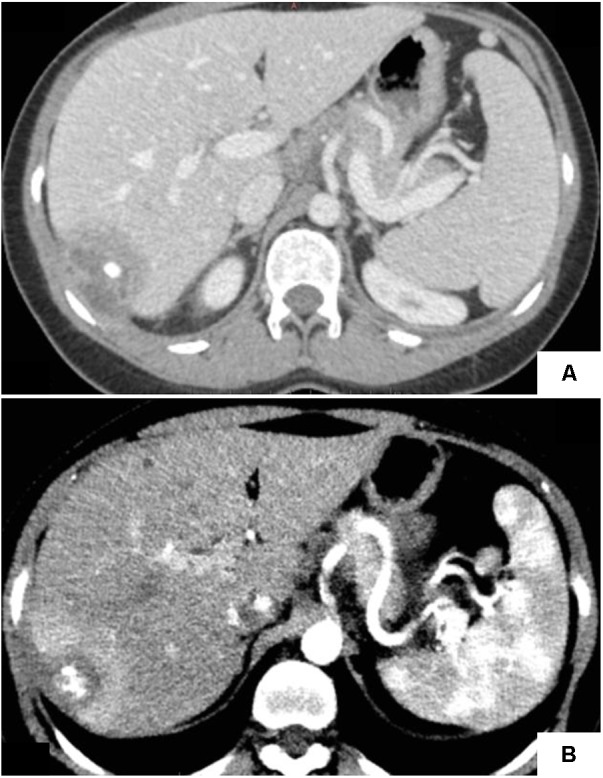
Abdominal CT-scans showing liver abscess. (A) Central calcification of the liver abscess observed in 2010, suggesting of a granulomatous infection. (B) The abscess observed in 2016 showing heterogeneous density and reduced size as compared to 2010.
